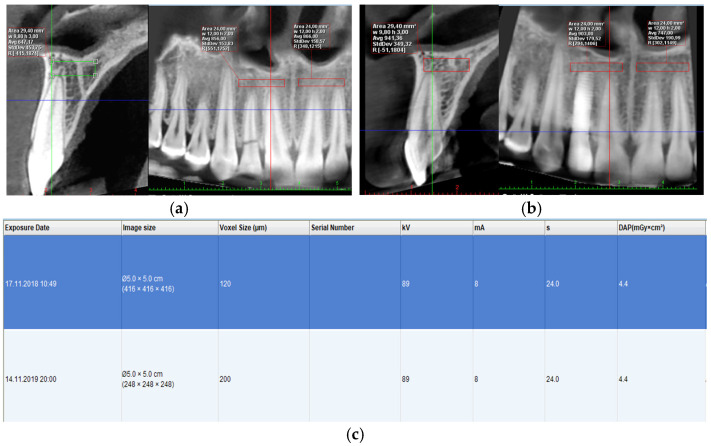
Initial CBCT view, definition of area of interest (a); post-surgical CBCT appearance after 12 months, same area of interest (b); parameters of acquisition of both CBCTs (c).
